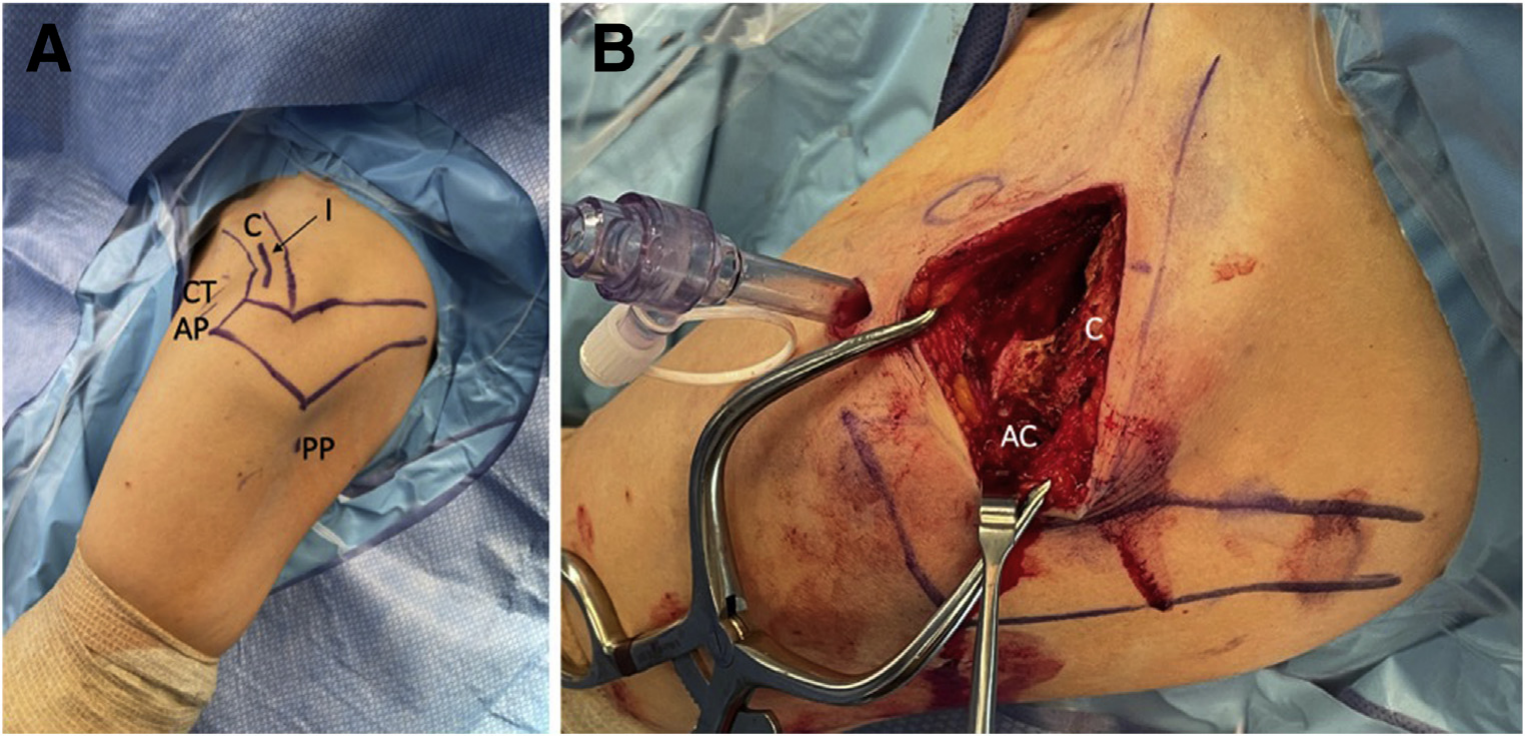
(A) A left shoulder is shown in the beach chair position. Shoulder landmarks have been marked including the clavicle (C), the tip of the coracoid (CT), the AC joint (AC), the anterior arthroscopic portal (AP), the posterior viewing portal (PP), and the planned incision (I) over the clavicle. (B) The same left shoulder is shown with the clavicle (C) and AC joint (AC) exposed through an incision down the longitudinal axis of the clavicle. Blunt dissection has been carried out just off the anterior edge of the clavicle down to the base of the coracoid (not pictured). Anterior and posterior arthroscopy portals also have been established.
